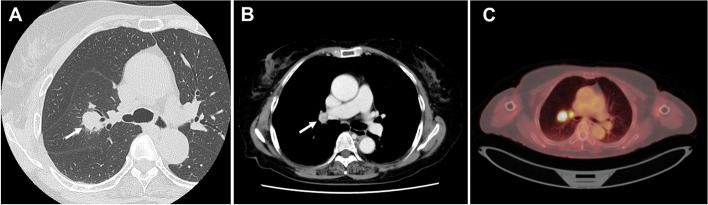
A Chest CT scan using the lung window setting showing a pulmonary nodule measuring 23 mm in diameter in the right upper lobe (arrow). B Contrast-enhanced CT scan using the mediastinal window setting showing hilar lymph node (station 11s) enlargement (arrow). C PET/CT scan showing 18F-FDG uptake in the tumor and in the hilar lymph nodes (station 11s). CT: computed tomography, PET: positron emission tomography, 18F-FDG: 18-fluorine-fluorodeoxyglucose
